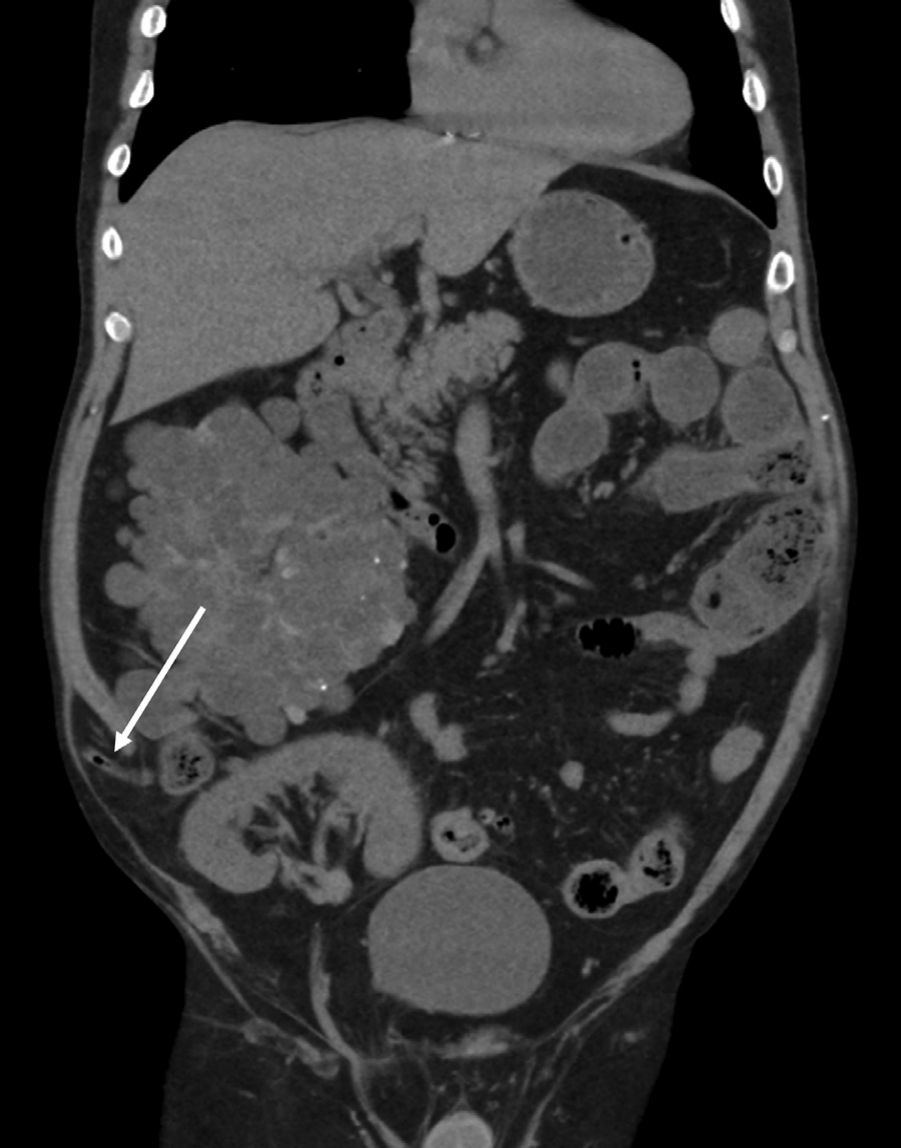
Prior CT abdomen and pelvis without intravenous contrast (performed 6 months prior to presentation) demonstrates presence of vermiform appendix (white arrow) in an incisional hernia sac. Of note: The image also includes, right polycystic kidney and transplanted kidney in right Iliac Fossa.
